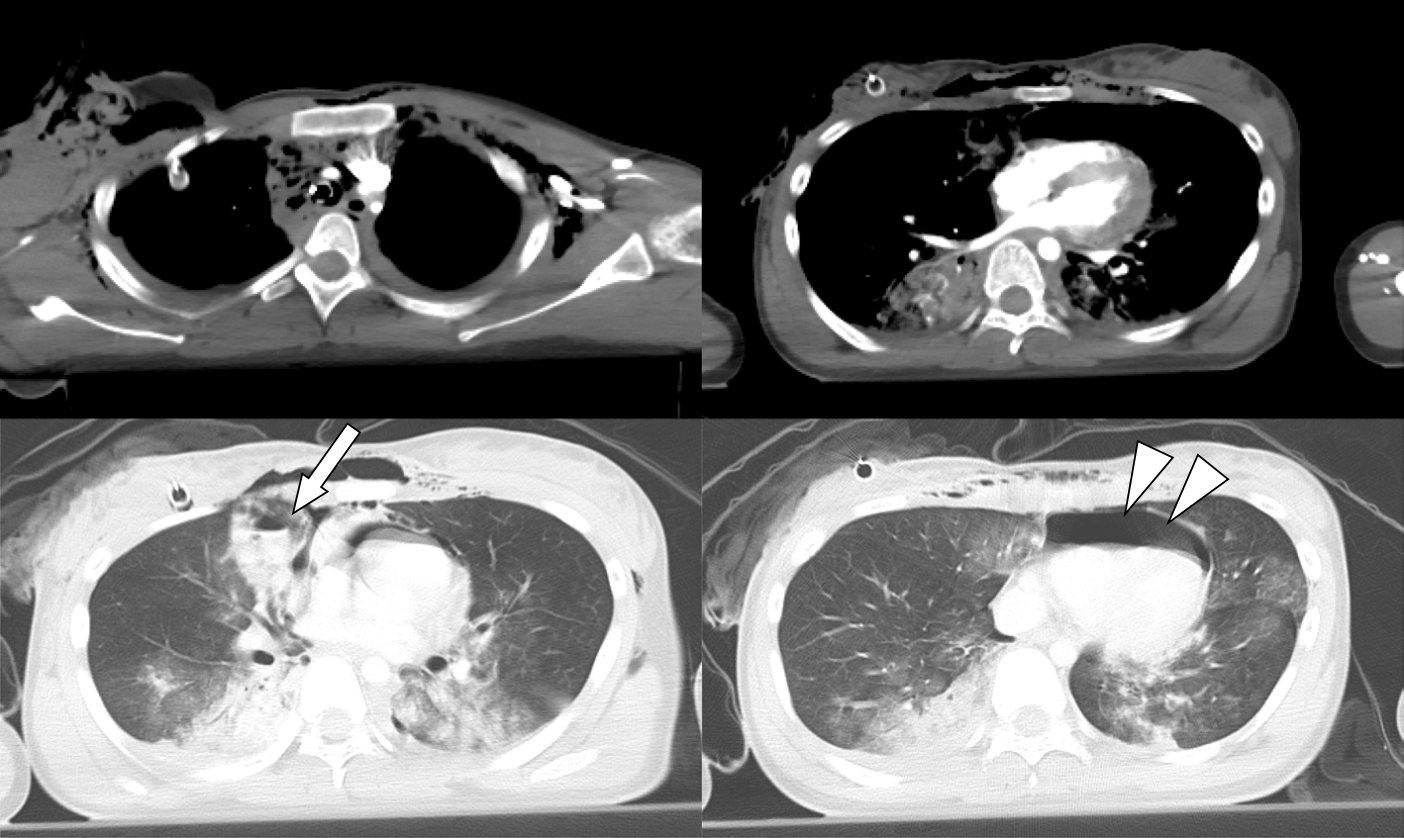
Computed tomography findings on admission. Computed tomography shows an intraparenchymal laceration of the right middle lobe (arrow) and pericardial emphysema (arrowheads)
